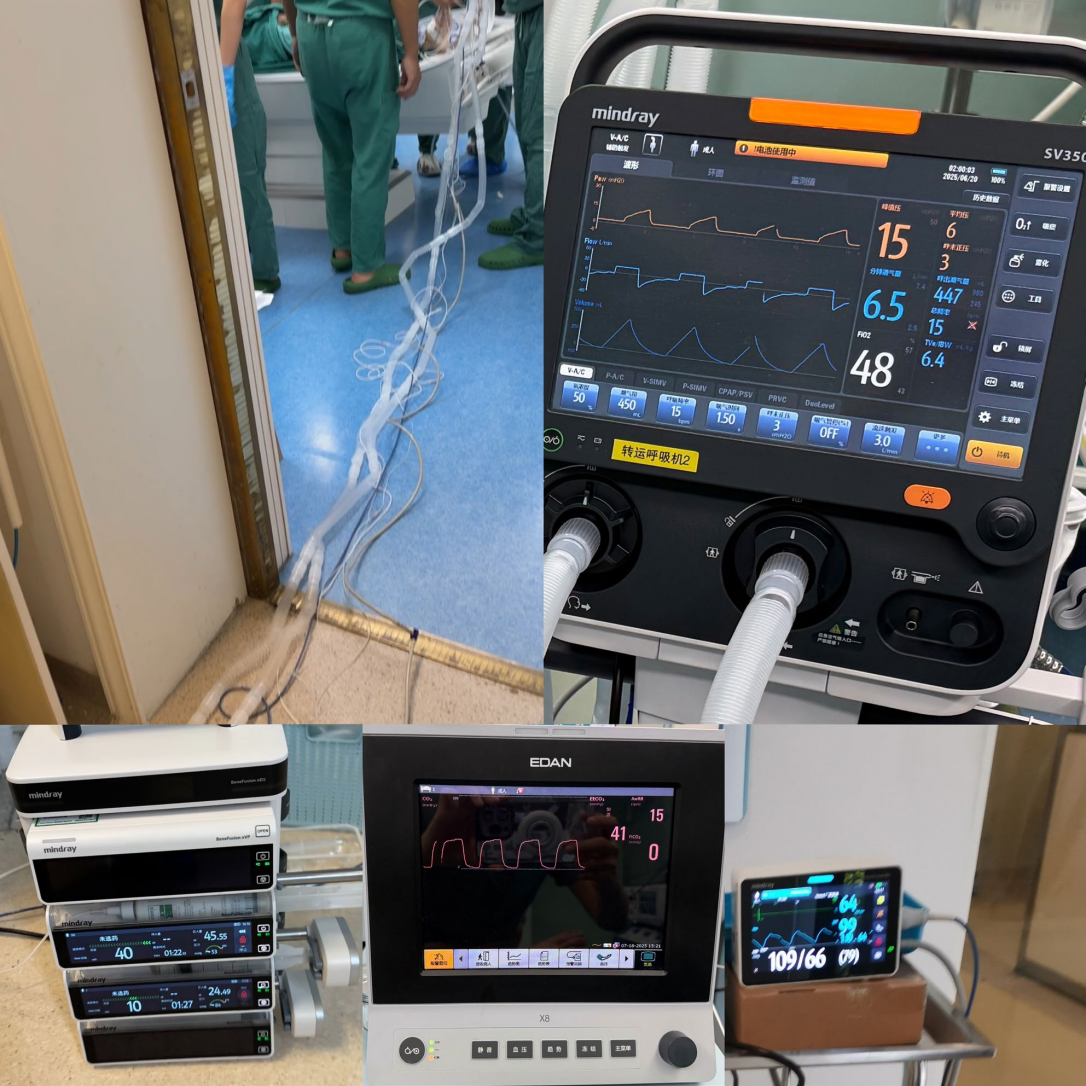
The transport ventilator (Mindray SV350), infusion pumps, and the monitoring of EtCO2 (EDAN X12), SpO2 and NIBP (Philips IntelliVue MX800) were placed outsidethe MRI suite. The upper part of the figure, the extended tubing and circuits connected with patient in a linear manner
